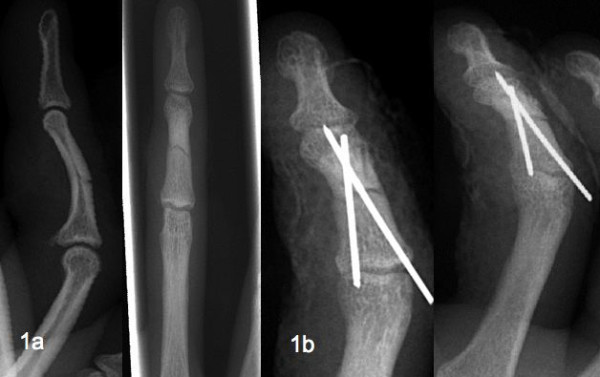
Initial X-ray after injury (a) and control X-ray after the osteosynthesis (b).
